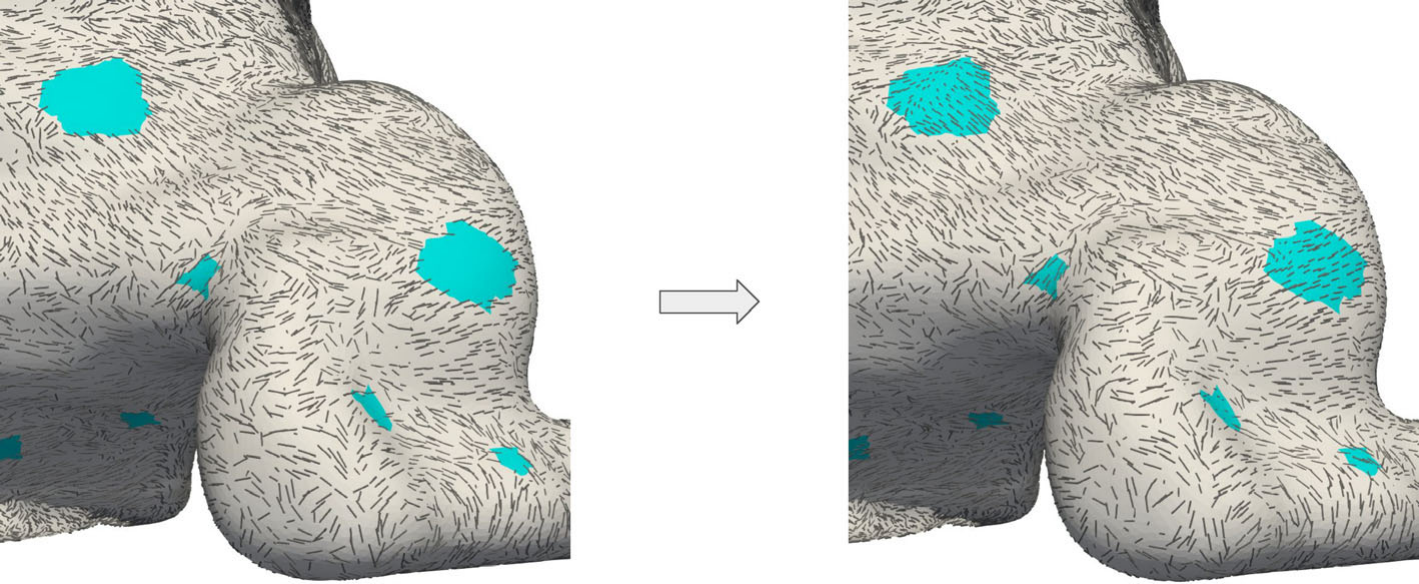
Recovering the left‐atrium fiber field on the epicardium using the harmonic‐extension algorithm: on the left, the field as reconstructed from DT‐MRI data where the regions with missing data are colored in light blue; on the right, the recover field. The data is taken from^86^
